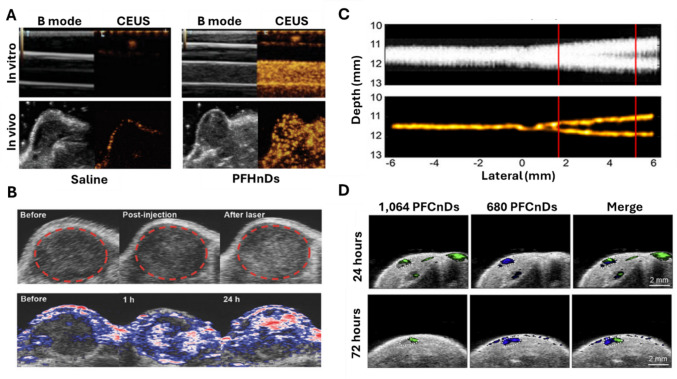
Imaging applications of PFCnDs. A Contrast-enhanced ultrasound (CEUS) with PFCnDs: representative B-mode and CEUS images demonstrate robust ultrasound contrast generation by PFCnDs in vitro and in vivo. Reproduced with permission from [67]. B Extravascular tumor imaging with PFCnDs: the nanoscale size of PFCnDs facilitates extravasation from tumor vasculature and accumulation within tumor tissue, enabling ultrasound imaging of extravascular tumor regions following vaporization. Reproduced with permission from [75]. C Super-resolution ultrasound imaging with PFCnDs: Blinking PFCnD signals yield sparse localizations that can be temporally accumulated to reconstruct microvascular structures beyond the conventional diffraction limit. Reproduced with permission from [83] Copyright 2021 American Chemical Society. D Multiplexed lymphatic imaging with PFCnDs: color-coded PFCnD formulations enable multiplexed ultrasound and photoacoustic imaging to distinguish multiple agents while supporting longitudinal visualization of lymphatic structures and drainage pathways. Reproduced with permission from [80]
